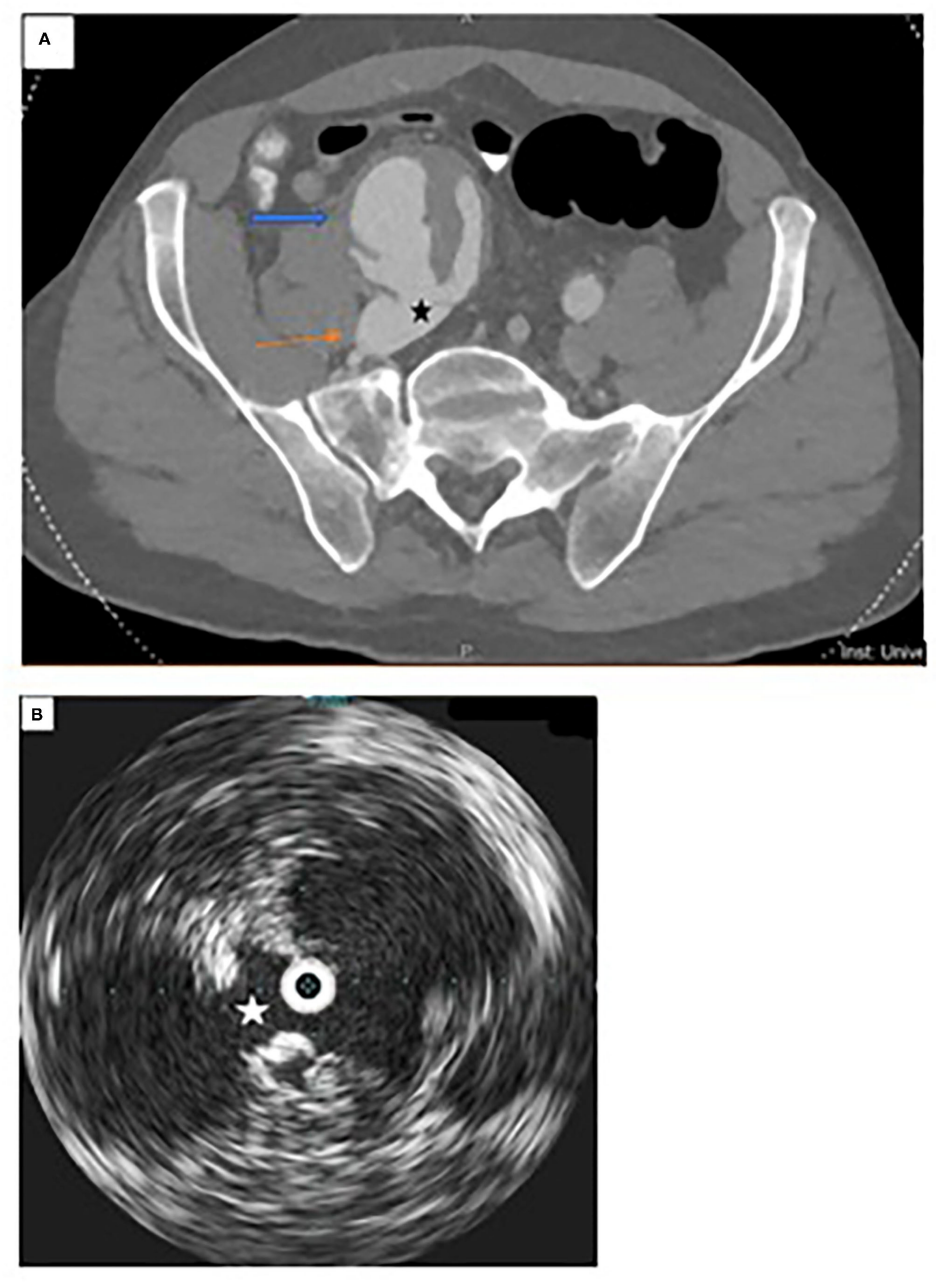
Additional imaging of fistula. (A) CT angiogram demonstrating arterial-venous fistula between the common iliac artery (blue arrow) and common iliac vein (orange arrow). (B) Intravascular ultrasound demonstrating arterial-venous fistula between the common iliac artery and common iliac vein.
